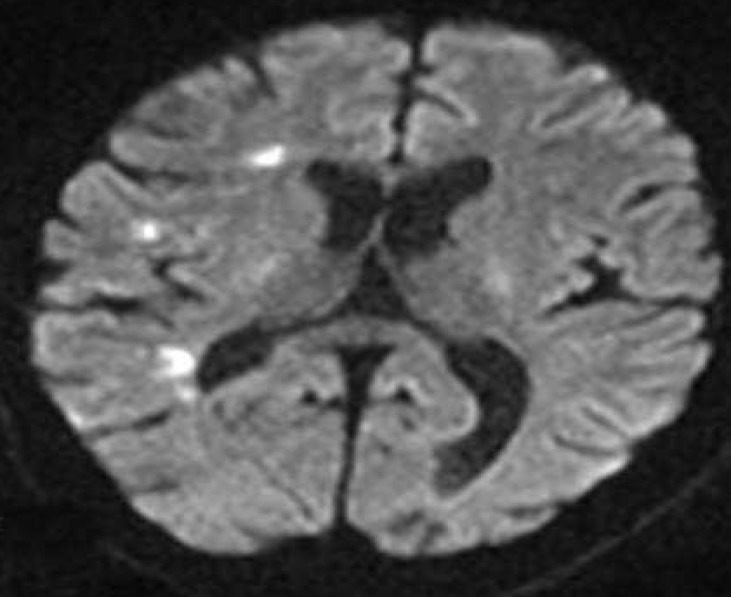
Diffusion weighted magnetic resonance imaging revealed multiple new lacunar infarcts in subcortical white matter of right cerebral hemisphere
